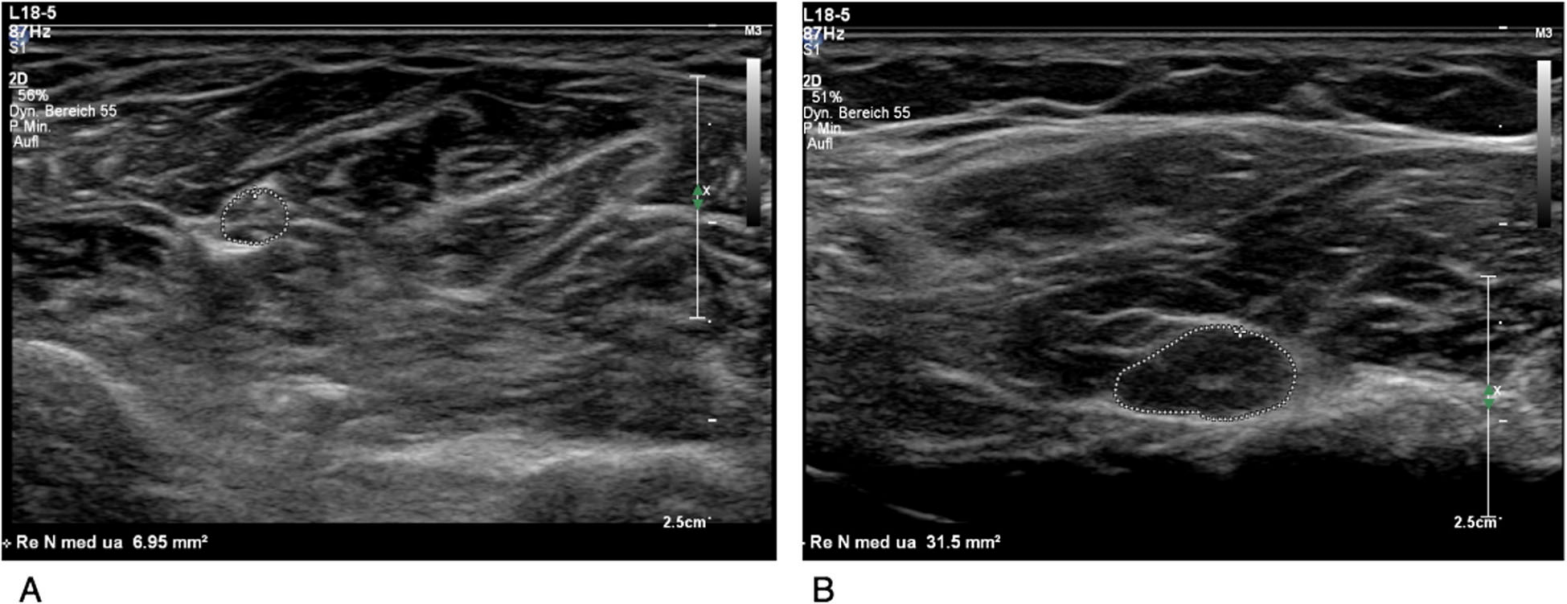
a Normal median nerve at the middle of the forearm between the flexor digitorum profundus and superficialis muscles with a normal CSA of 6.95 mm^2^ and normal fascicular structure. b Significantly enlarged median nerve at the forearm with a CSA of 31.5 mm^2^ in a patient with CIDP. Some swollen fascicles and a hypoechoic structure can be depicted. Ultrasound stetting except focus are the same in both images
